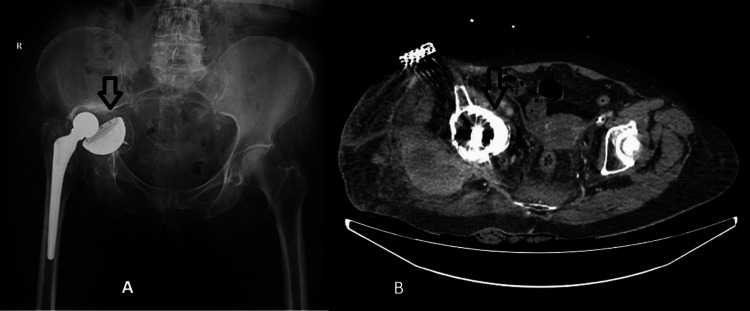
A. Preoperative antero-posterior pelvis X-ray. B. Preoperative pelvis CT-scan-axial view.
